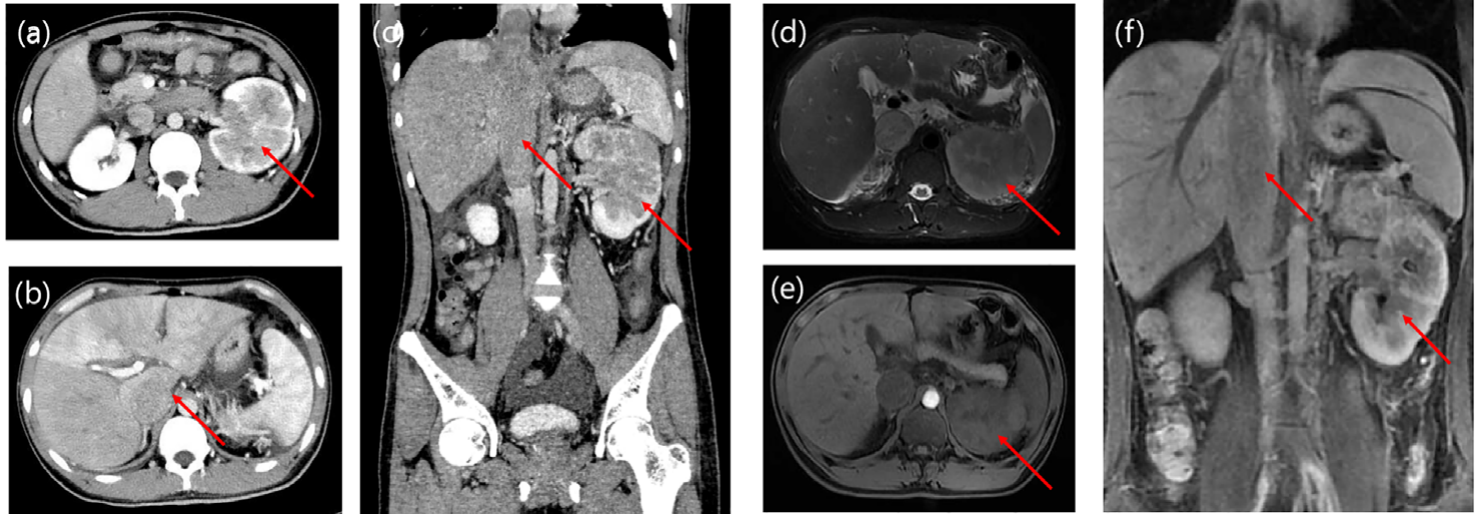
Enhanced CT (A–C) and MRI (D–F) imaging. Significant abnormal findings of left kidney and tumor thrombus of vena cava were noted *(arrow)*.
